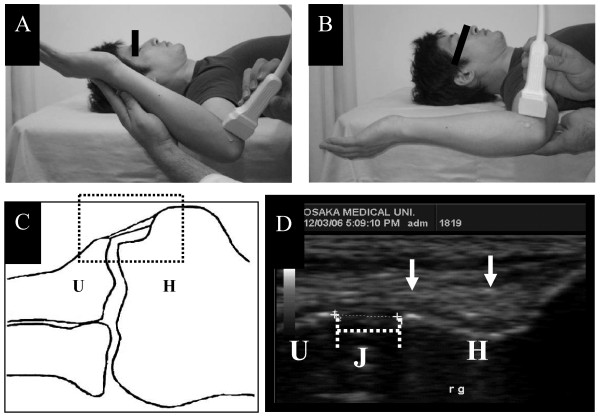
Ultrasonography of the elbow (A) without gravity stress (shoulder at 60° of external rotation) and (B) under gravity stress. (C) Sketch and (D) ultrasonogram (region encompassed by dotted box in C) of the medial elbow. Arrow, Anterior bundle of the ulnar collateral ligament; H, humerus; U, ulna; J, joint space (dotted line).
